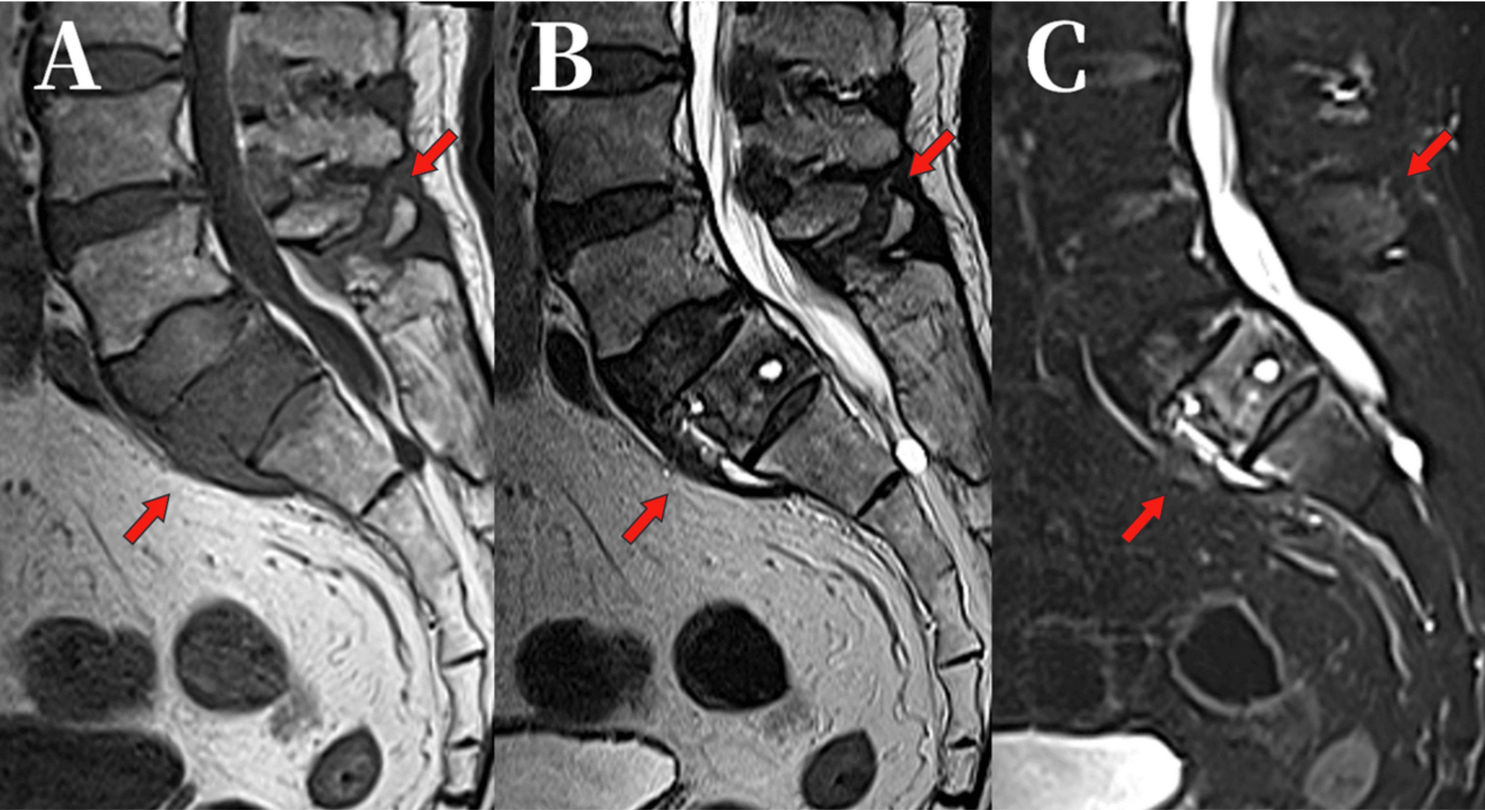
Sagittal MRI images. (A) T1-weighted (B) T2-weighted (C) STIR showing persistent signal changes within the vertebral body without improvement (12 weeks after injury). MRI: magnetic resonance imaging, STIR: short tau inversion recovery.
